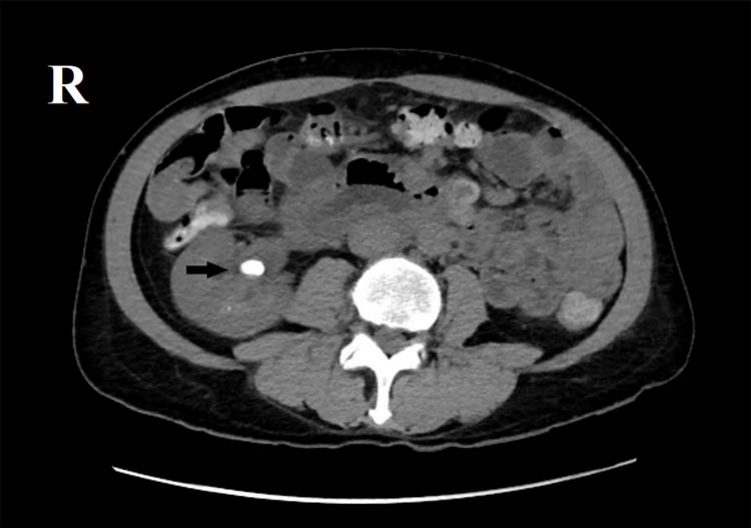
CT Scan of abdomen showing right renal pelvis calculus.
